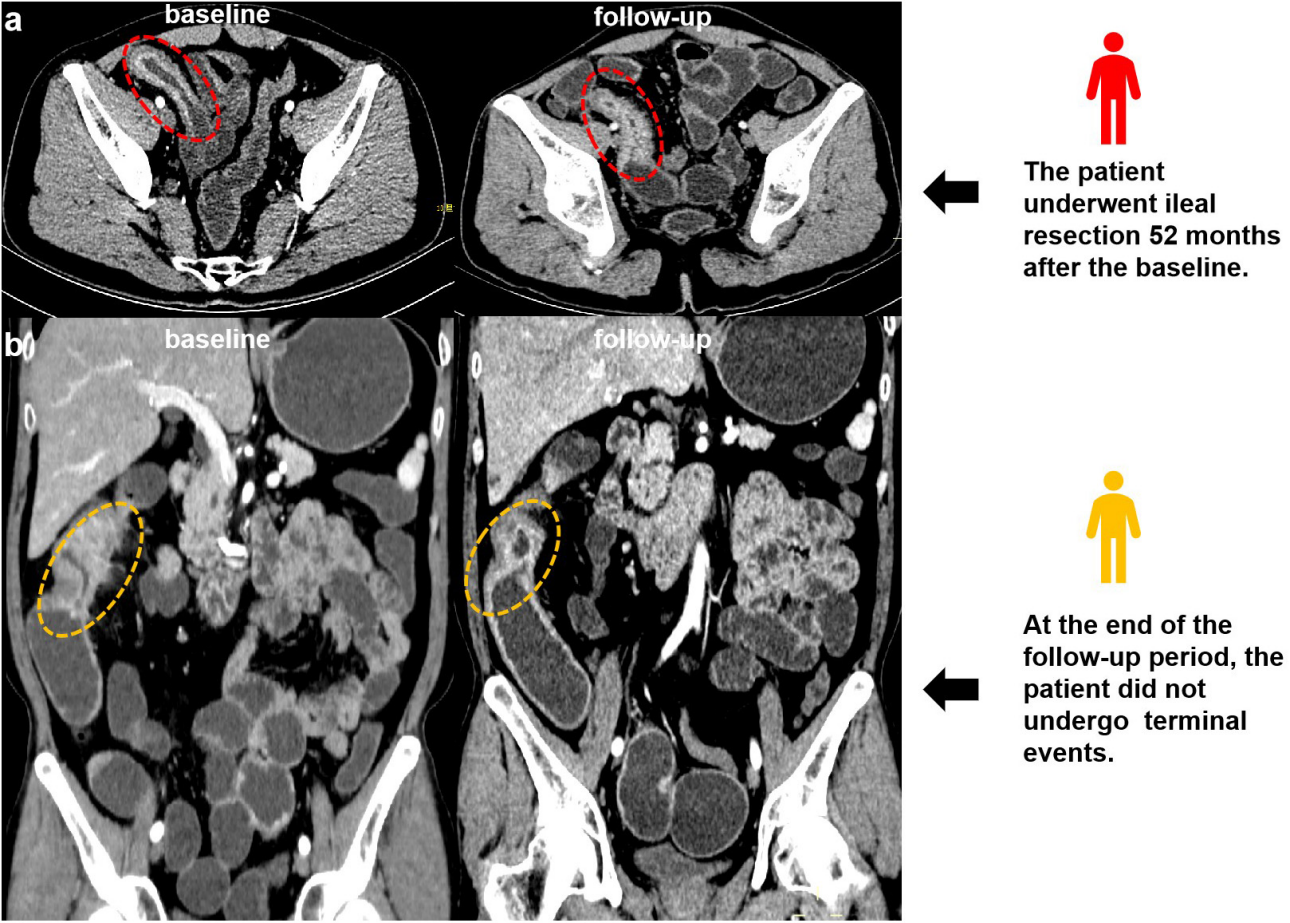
(a) Examples of imaging: A 35-year-old man with small bowel stricture and the wall thickening is observed in the baseline and follow-up (red circle). The patient underwent ileal resection 52 months after the baseline computed tomography enterography (CTE). (b) Examples of imaging: A 49-year-old man with small bowel stricture and the wall thickening is observed in the baseline and follow-up (yellow circle). At the end of the follow-up period, the patient did not undergo endpoint events.
